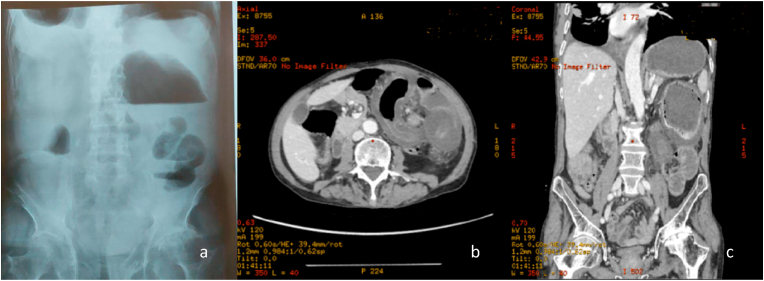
(a) Plain X-ray of the abdomen in erect posture with an air fluid colonic level in the left hypochondrium (b) CT SCAN showing the whirl sign (c) CT scan showing the dilated caecum in the left hypochondrium.
